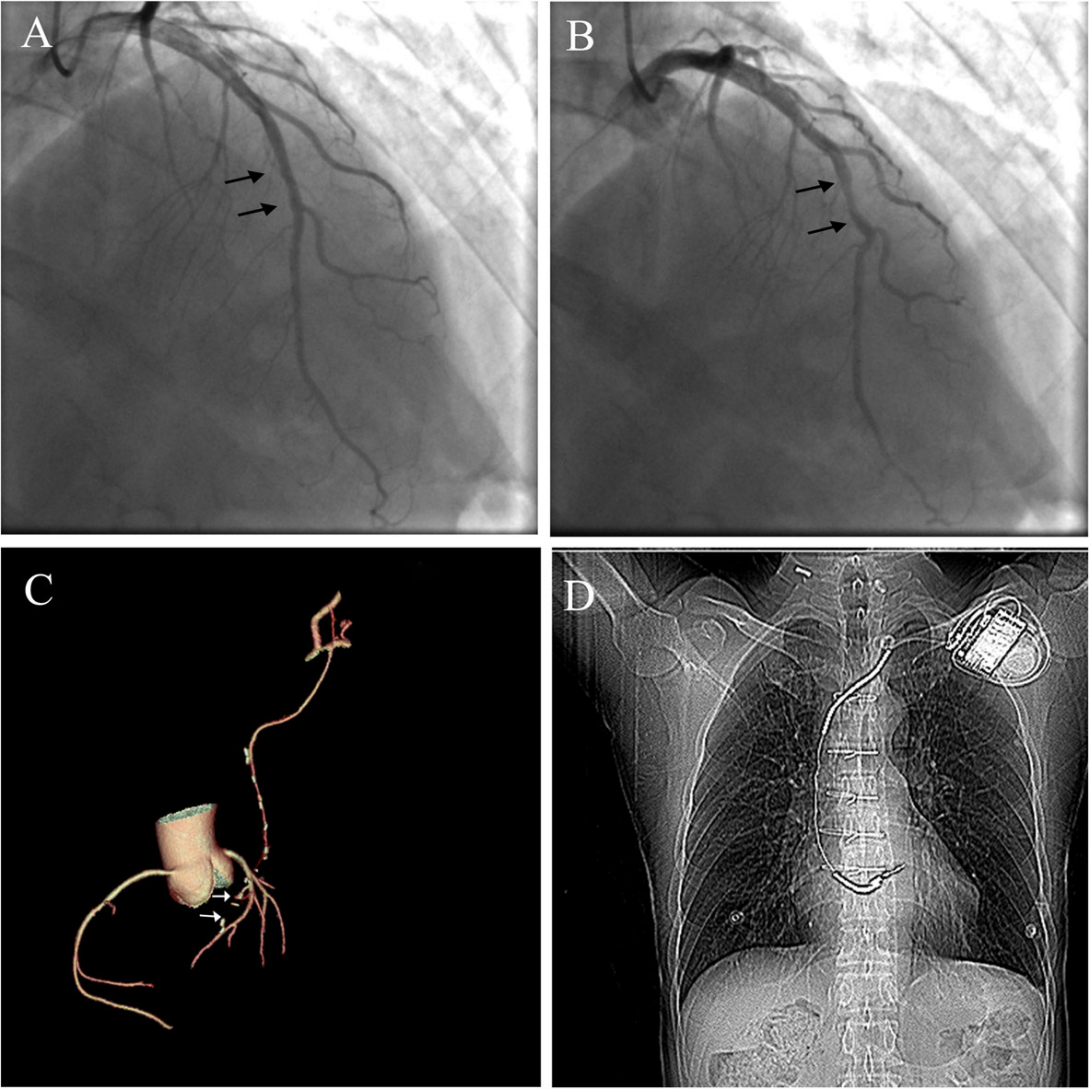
RAO cranial view of the LAD at baseline displaying MB segment during systolic period (a black arrows), and diastolic period (b black arrows); volume-rendered 3-dimensional reconstruction displaying occlusion at the end of the LIMA (c white arrows); a chest radiograph showing the ICD device and its lead location d
